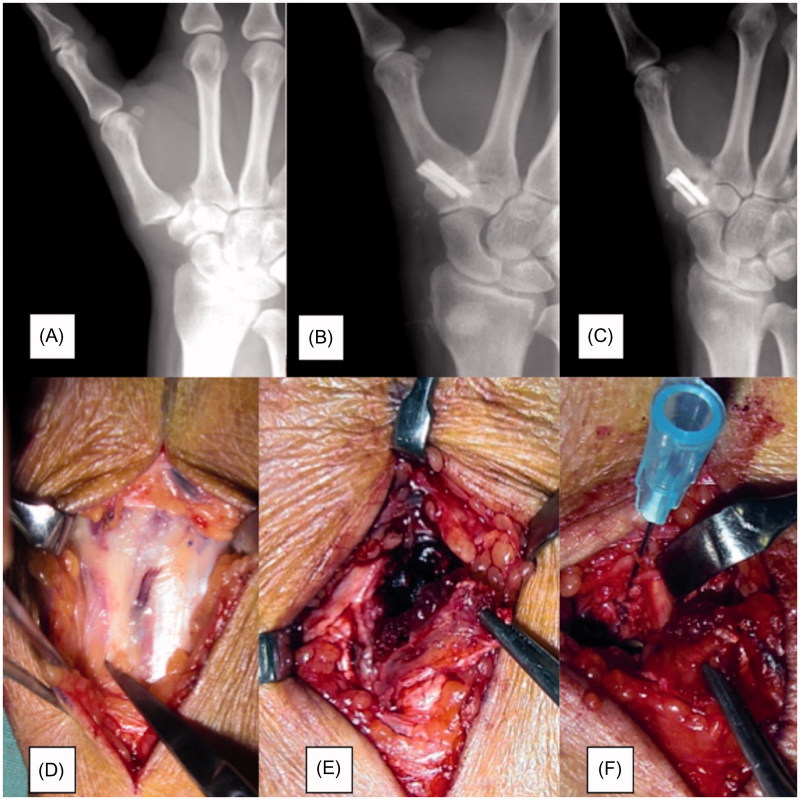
Case 1: a 58-year-old female. (A) Preoperative radiograph (dorsoplantar view) of the right hand showing dislocation of the first carpometacarpal joint. (B) Immediately postoperative radiograph after the first surgery. (C) Radiography at 6 months after the first surgery showing nonunion. (D) Intraoperative photograph of the second metacarpal artery. (E) Photograph of the vascularized bone graft at the time of harvesting. (F) Photograph of the vascularized bone graft at the time of transferal to the first carpometacarpal joint.
